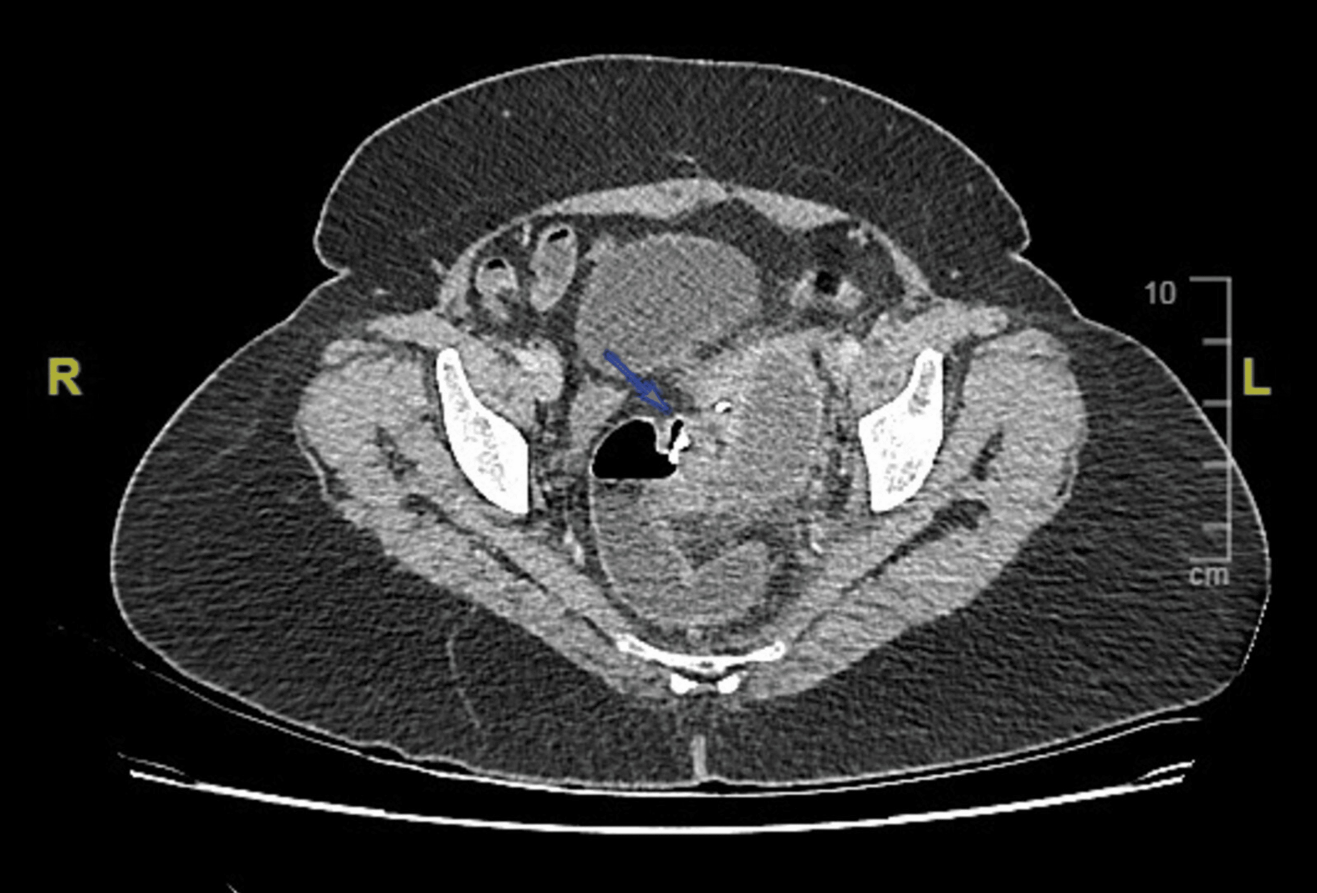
Axial section of CT of the abdomen showing sealed-off sigmoid colon perforation with a foreign body.
